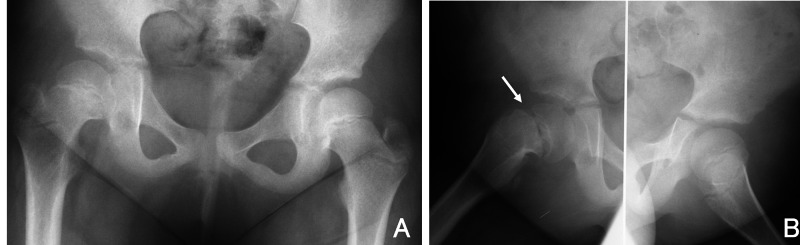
Preoperative imaging (5 years 3 months old). (A) Anteroposterior and (B) Rauenstein radiographs of the bilateral hip show a posterior-inferior slip of the femoral epiphysis of the right hip (arrow).
